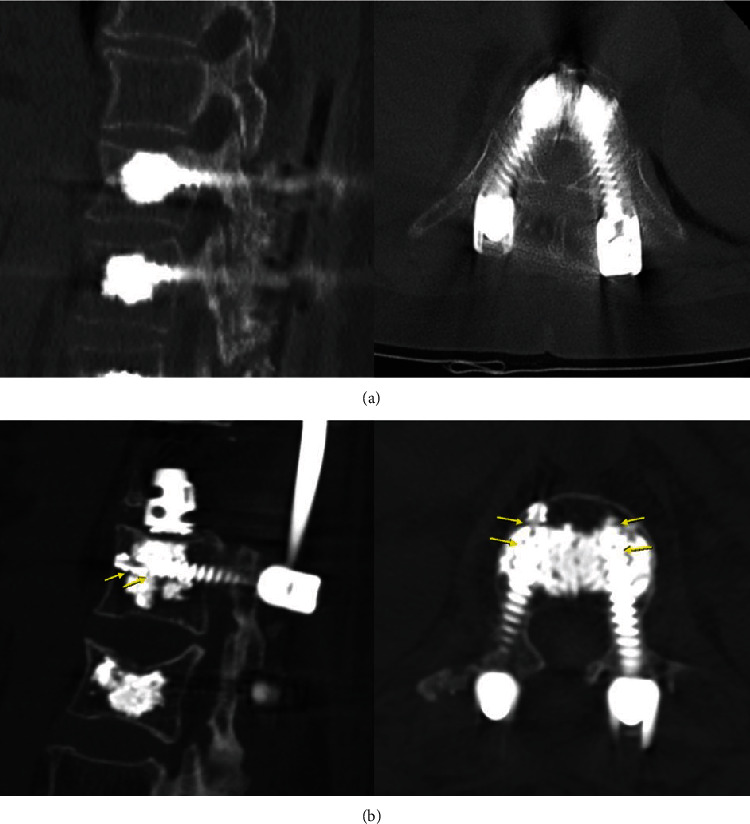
Cement distribution pattern of traditional cement method and pressurized method confirmed in clinical computed tomography (The guide arrow indicate the side hole of fenestrated screw.).
